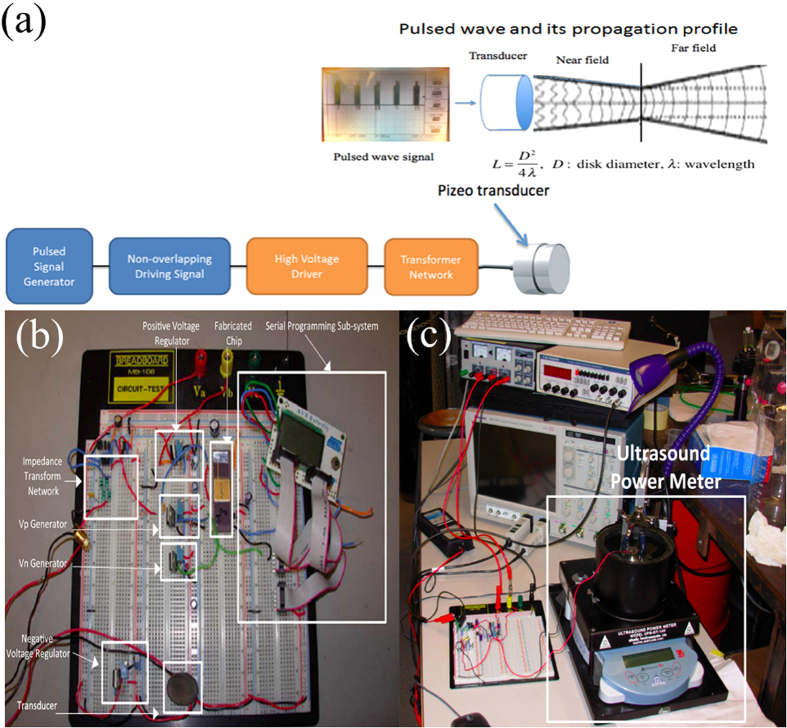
(a) LIPUS design consists of various modules: such as pulsed signal generation, signal amplification and impedance matching for driving piezoelectric transducers, (b) the detailed LIPUS design was mapped on a breadboard with individual components marked, (c) the ultrasound power meter was used to calibrate the output intensities of pulsed wave.
